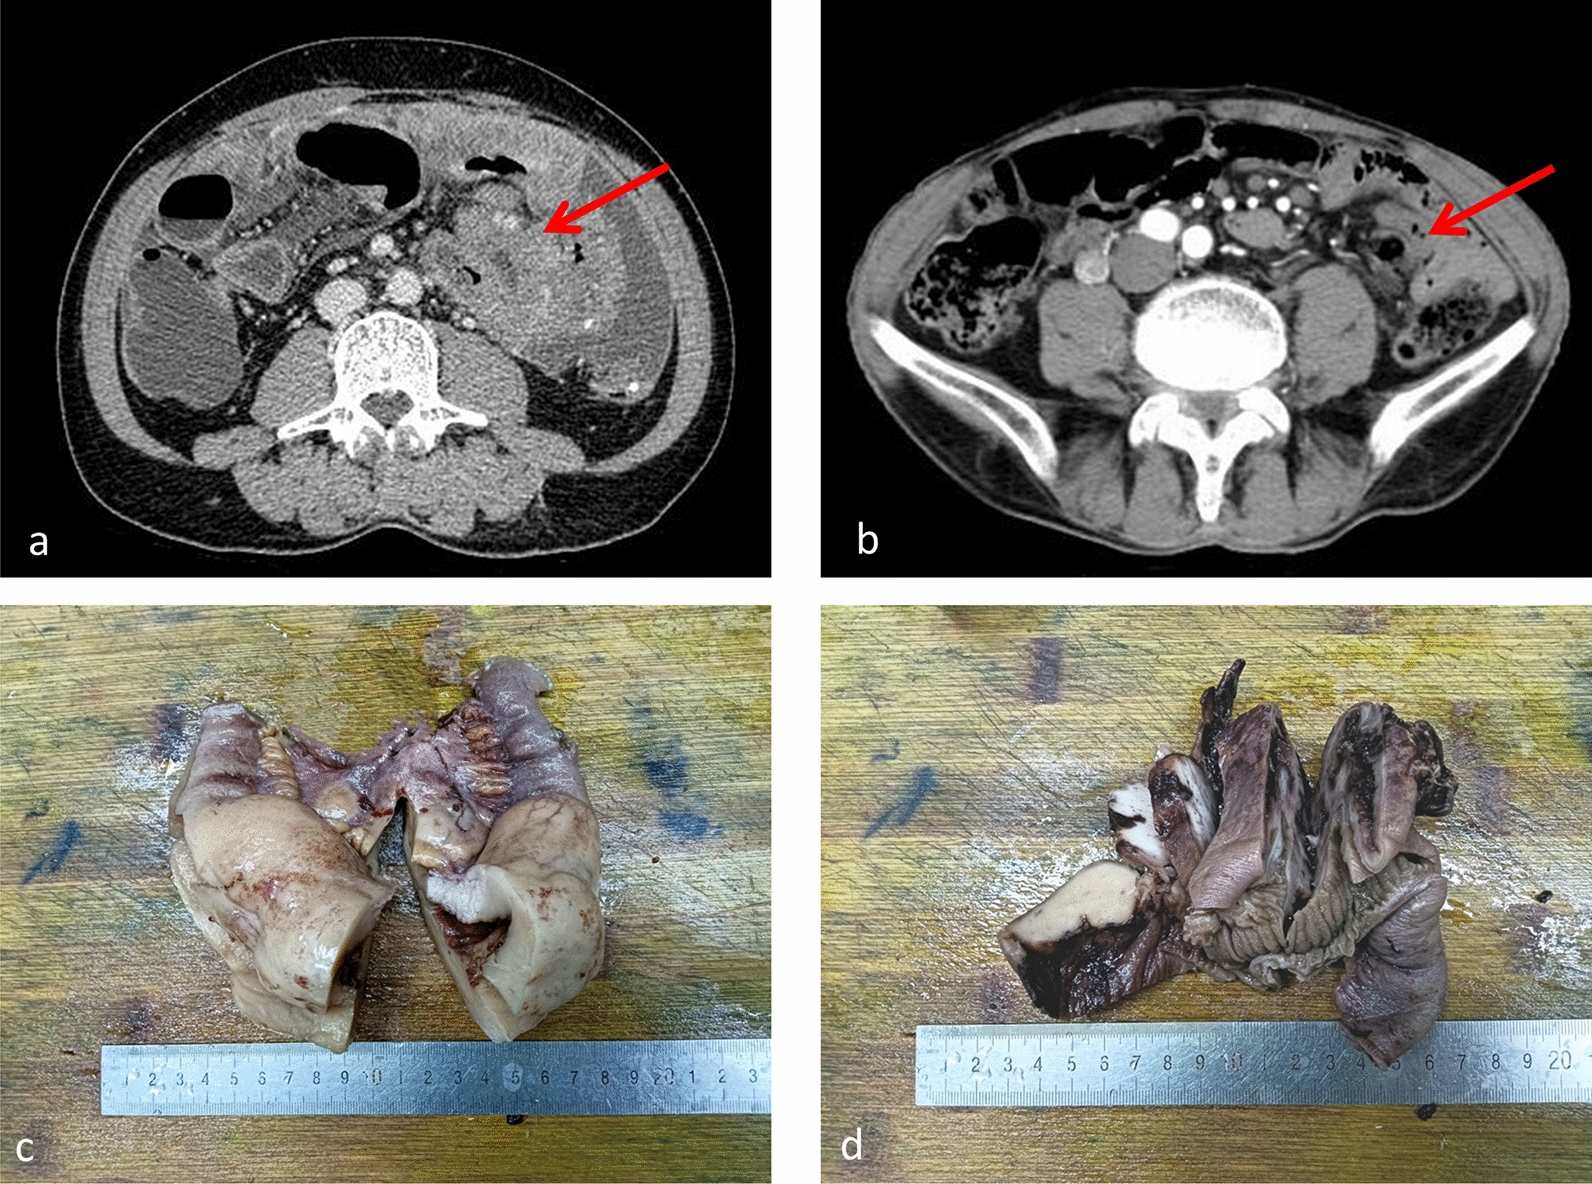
a CT showing small bowel occupying lesion; b CT showing small bowel occupying with adhesions; c large body showing small bowel mass; d large body showing small bowel mass
